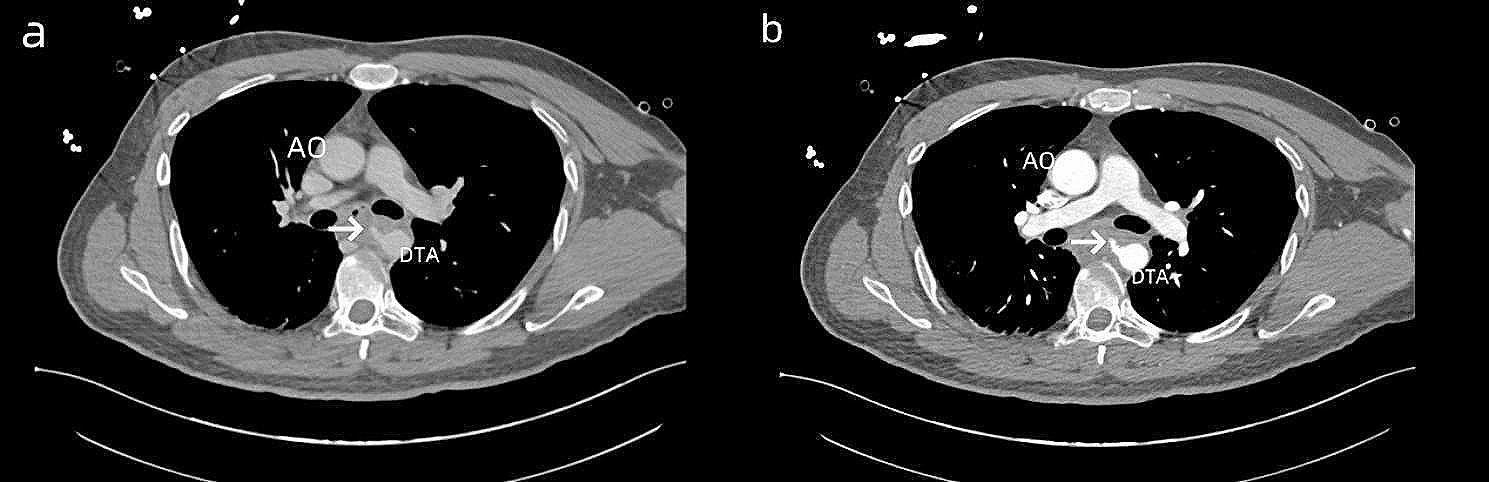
(a, b) Preoperative computed tomography images. CT showed the foreign body (white arrow) Ao: aorta, DTA:Descending Thoracic Aorta
